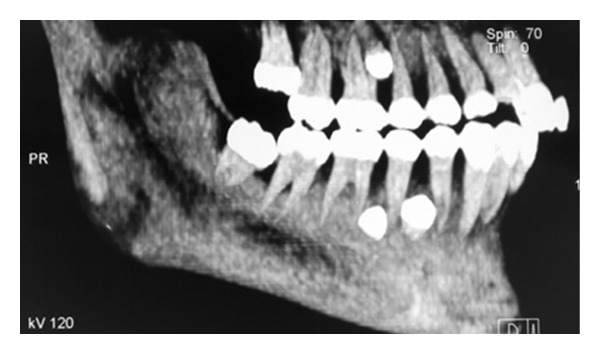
CT scan view of the right side showing three supernumeraries.
